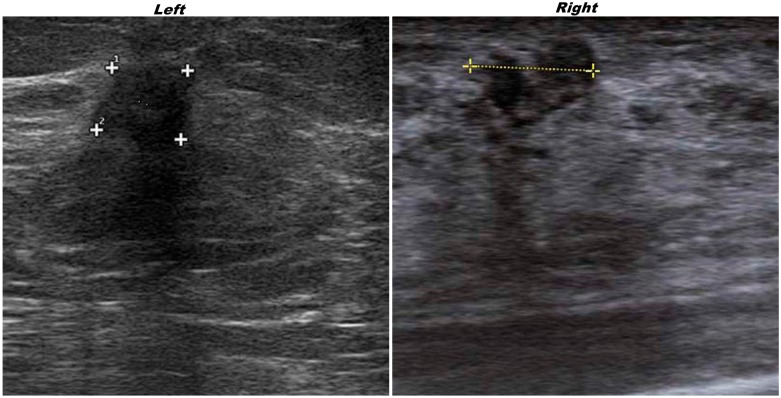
Ultrasonography of the left invasive ductal carcinoma(IDC) and right granular cell tumor (GCT) breast lesions. “Plus signs” underline the presence of suspicious lesions.
